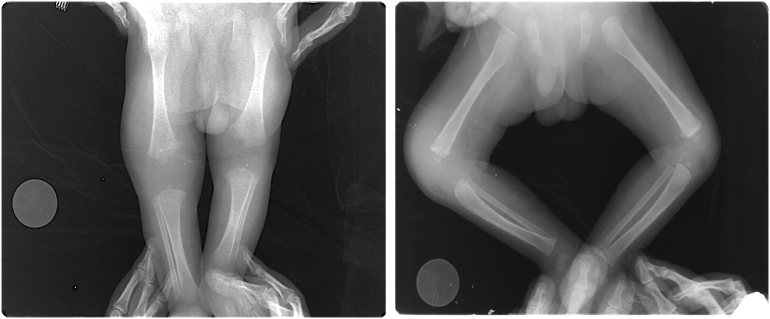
A 1-month-old boy radiograph X-ray of bilateral knee joint with septic arthritis of the right knee joint.
